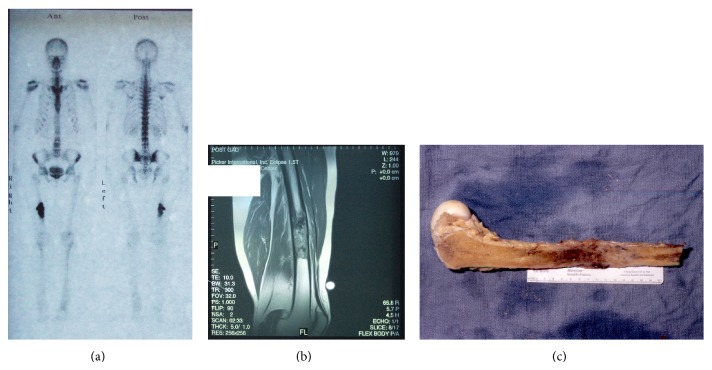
Osteosarcoma. (a) Whole body scan shows abnormally intense uptake of the radionuclide (Tc) within the middiaphysis of the right femur. No increased radionuclide uptake is seen anywhere else in the bony skeleton. (b) MRI scan displays an extensively abnormal signal in the diaphysis of the right femur. It is surrounded by soft tissue involvement. (c) Bone lesion displayed postoperatively.
